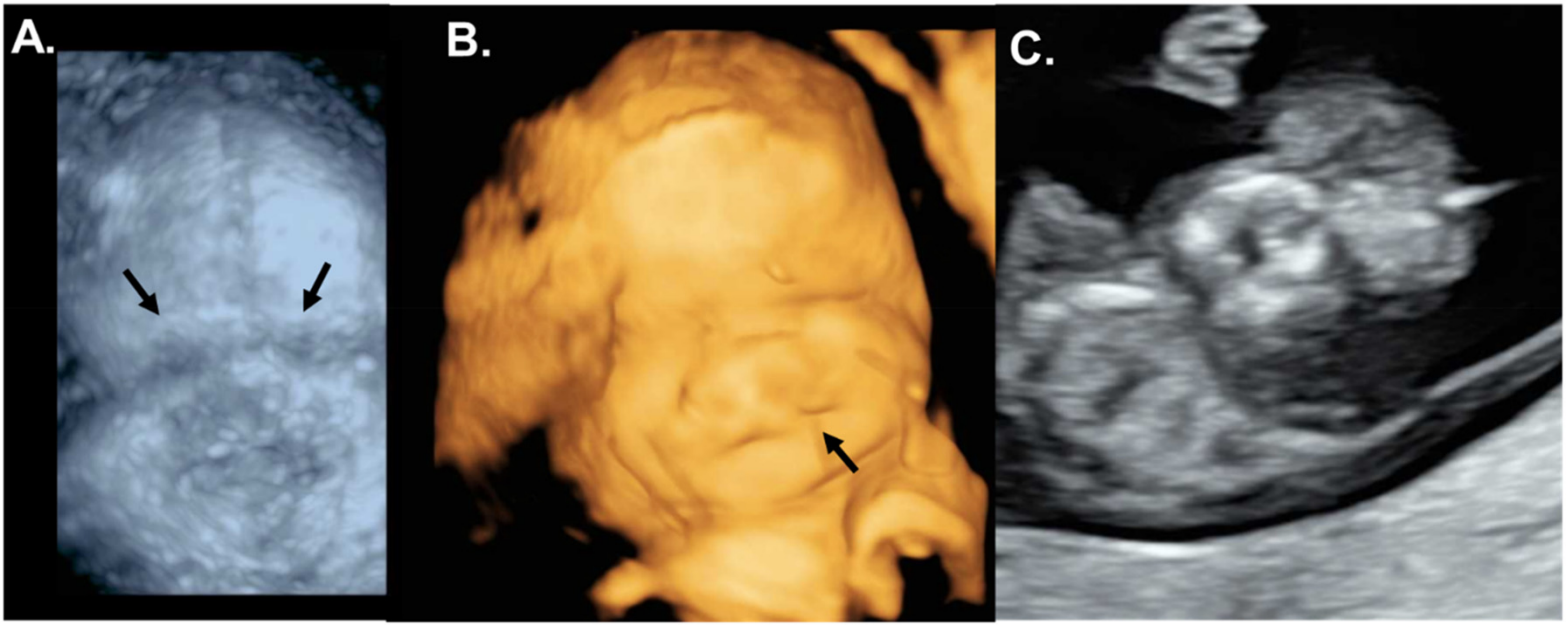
Antenatal ultrasounds showing the facial characteristics at different stages of pregnancy. (A) 3D static ultrasound (skeletal mode) of the skull in frontal view, with the missing representation of the orbits (marked with arrows) at 32 + 4 weeks of gestation. (B) 3D surface view of the face showing conspicuously low-lying eyelids and a cleft lip (marked with an arrow) at 32 + 4 weeks of gestation. (C) 2D frontal view of the fetal facial skull during first-trimester screening, with missing orbits at 11 + 2 weeks of gestation in the subsequent pregnancy.
